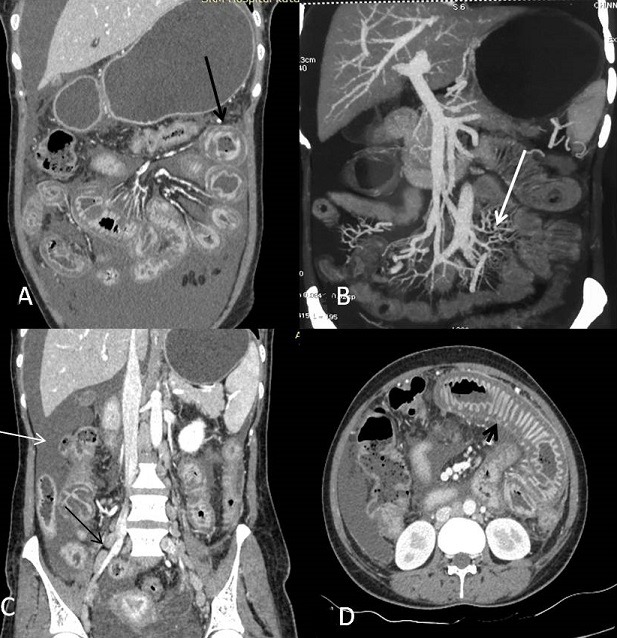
(A) coronal Contrast CT showing target sign (black arrow) with enhancing mucosa and serosa/muscularis propria with diffuse hypoattenuation of submucosa; (B) showing increased vascularity of adjacent mesentery (white arrow); (C) showing retroperitoneal lymphadenopathy (thin black arrow) and ascites (thin white arrow); (D) showing fold thickening of Jejunal loops(short black arrow)
